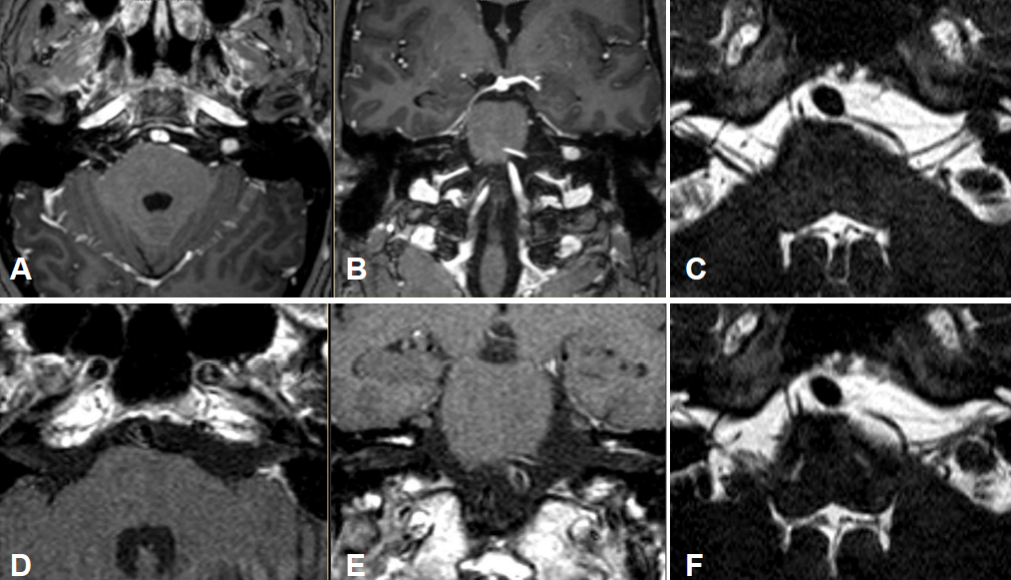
Upper row: preoperative MRI, T1-w + CM, A transversal; B coronal; C CISS transversal. Lower row: postoperative MRI, T1-w + CM; D transversal; E coronal; F transversal CISS.
